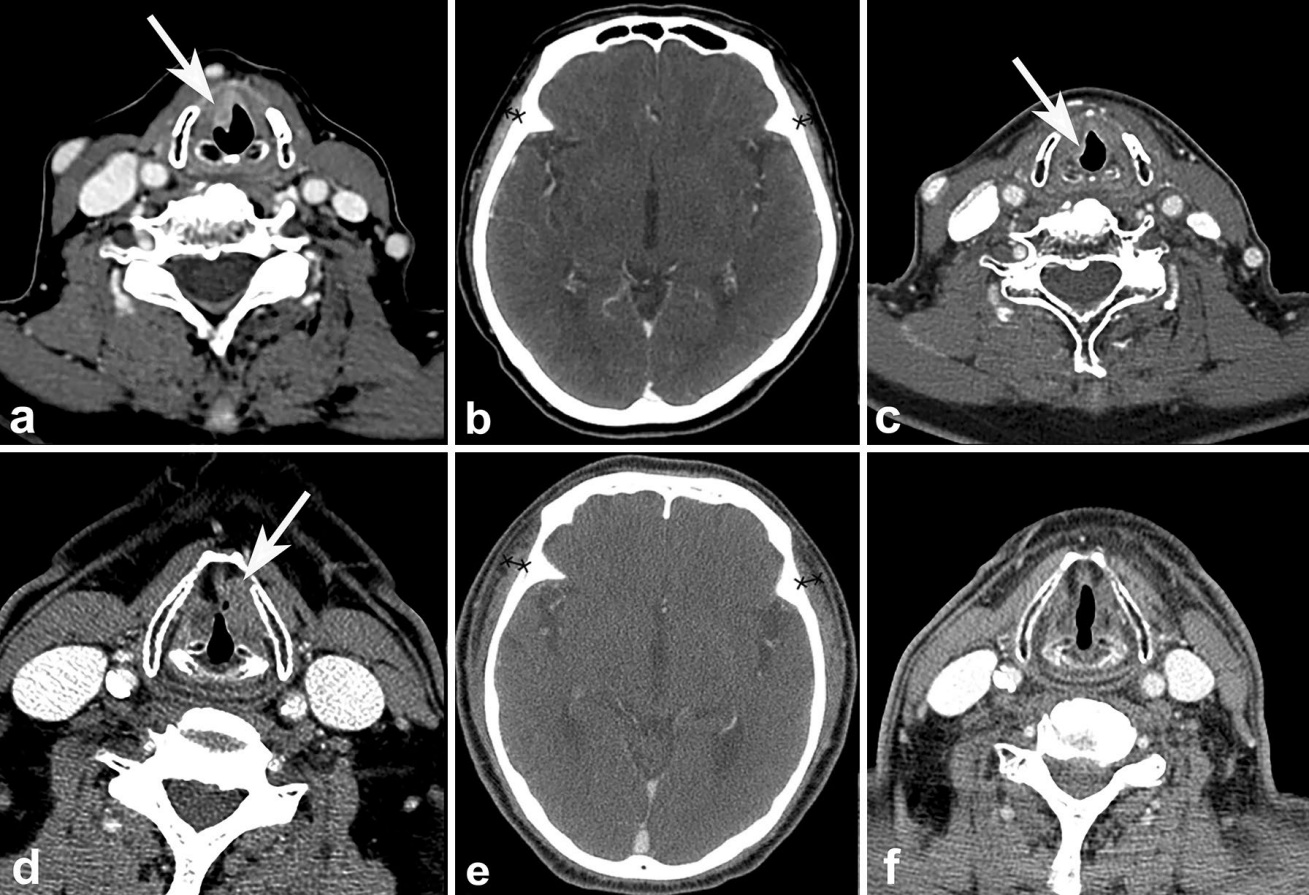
Representative cases of temporalis muscle thickness (TMT) on CT, and its association with progression-free survival. A 72-year-old female was diagnosed with glottic cancer (cT2N0M0) (a, arrow). On the axial CT scan, the averaged TMT was measured as 4.56 mm (b), which was less than the threshold of 6.24 mm. After 6 months after diagnosis, she developed local tumor recurrence in the anterior glottis (c, arrow). Another 74-year-old female who was diagnosed with glottic cancer (cT2N0M0) (d, arrow) had baseline TMT of 6.56 mm (e), which was larger than the threshold of 6.24 mm. During the follow-up period of 42 months, the patient did not develop tumor recurrence (f).
